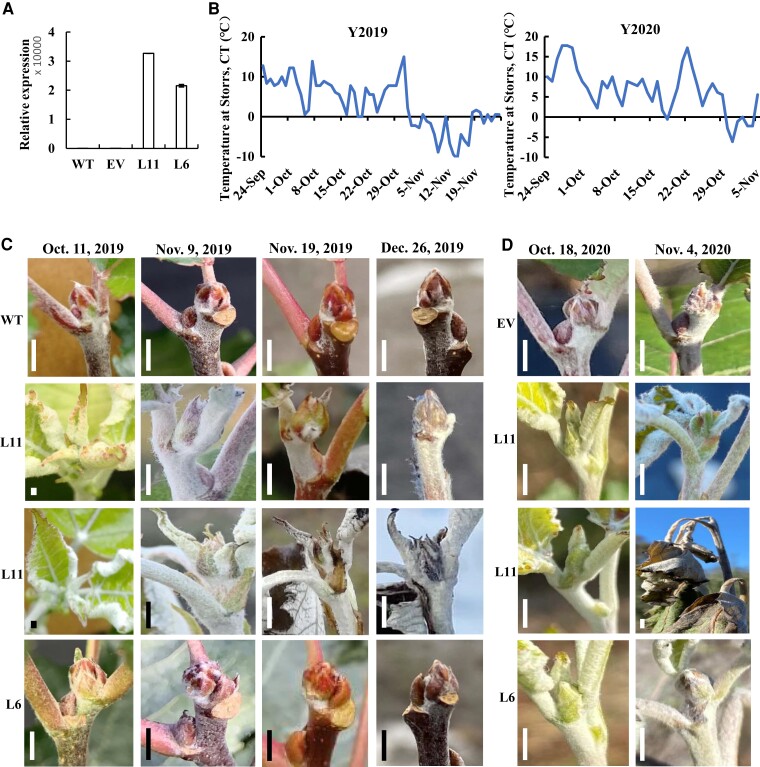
Heterologous expression of *AaRVE1* delayed seasonal dormancy in poplar in the field. A, Reverse transcription quantitative PCR (RT-qPCR) analysis to detect the expression of *AaRVE1* in transgenic plants expressing *AaRVE1* (L11, L6), empty vector (EV), and wild-types (WT). *Actin* gene was used as an internal control. Data are presented as mean ± Sd (*n* = 2). B, Lowest daily temperature in the field of Storrs, CT (41.8048° N, 72.2930° W). C and D, Apical phenotypes of the transgenic plants and WT in the field. Bar = 3 mm. In the year of 2019, all the L6 plants (9/9) showed slightly delayed dormancy compared with controls, while all the L11 plants (6/6) continued growing until early November when the temperature dropped below 0°C. The terminal bud of L11 gradually turned into dormancy-like phenotype in the middle of November. In the year of 2020, all the EV plants (6/6) turned into dormancy and stopped growing around October 18, while 42.9% (3/7) of L6 plants and 66.7% (4/6) of L11 plants continued growing until the snowfall and cold temperature (−6.1°C) on October 31.
